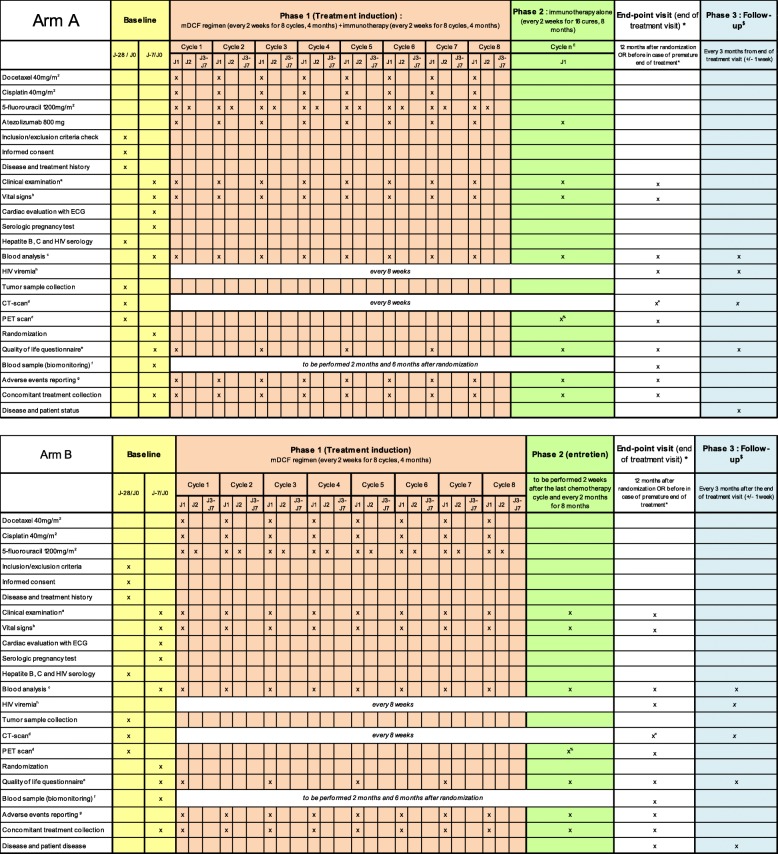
Schedule of enrolment, interventions, and assessments. a Clinical examination: height (at baseline only), weight, and ECOG-PS. b Vital signs: pulse, blood pressure and body temperature. c Blood analysis: complete blood count including red blood cells, haemoglobin, haematocrit, lymphocytes, white blood cells and differentials and platelets, blood electrolytes, bicarbonates, glycemia, proteinemia, albumin, blood urea nitrogen, creatinine, creatinine clearance (MDRD), calcium, magnesium, AST, ALT, gamma-GT, conjugated and total bilirubin, ALP, TSH, LDH, and C-reactive protein. d PET-scan at baseline, at first visit of phase 2 and at the end-point visit (except in case of early progression). CT-scans and PET-scans will be collected for a central review. e The EORTC-QLQC30 questionnaire: every second chemotherapy cycles in phase 1, and every 2 months during phase 2 until the end-point visit. f Blood samples for biomonitoring: 1 EDTA tube of 6 ml (plasma) and 8 EDTA tubes of 6 ml (PBMC). g According to the NCI-CTCAE guidelines version 4.03. h Only in HIV-positive patients. £ Immunotherapy alone: to be continued 1 cycle every to weeks up to a maximum of 12 months from the randomization date. * The end-point visit: to be performed 12 months after randomization or 4 weeks after the last chemotherapy cycle in case of premature end of treatment (for toxicity, progression, or patient or physician decision). $ The follow-up visit: to be performed every 3 months from the end-point visit to a patient death or at least 3 years after randomization
